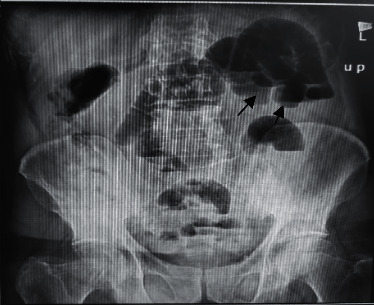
Male patient's upright abdominal X-ray.
